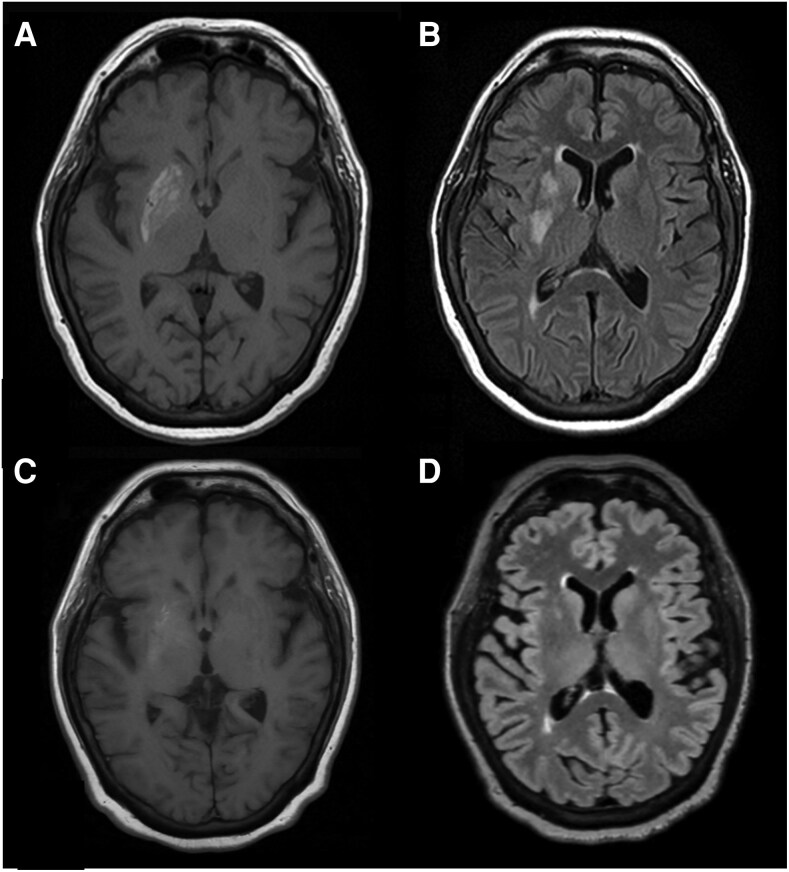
Magnetic resonance imaging of the brain demonstrating T1 hyperintensity (A) and increased signal on T2 FLAIR (B) of the right lentiform nucleus, with near-complete resolution of T1 signal (C) and full resolution of T2 FLAIR signal (D) on three-month follow-up imaging.
